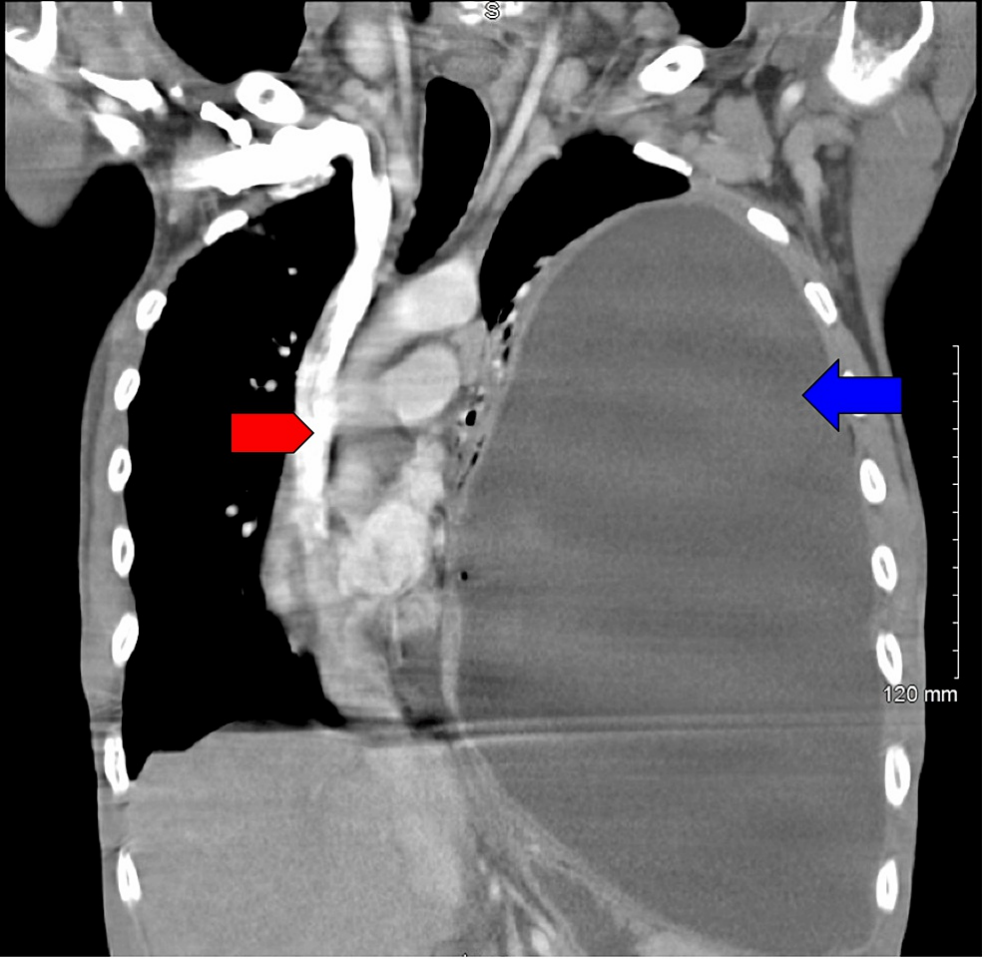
CT scan image (coronal) A coronal CT scan shows a considerable effusion of the left hemithorax (blue arrow) with significant mass effect upon the cardiac and mediastinal silhouette to the right with tracheal deviation (red pentagon).
